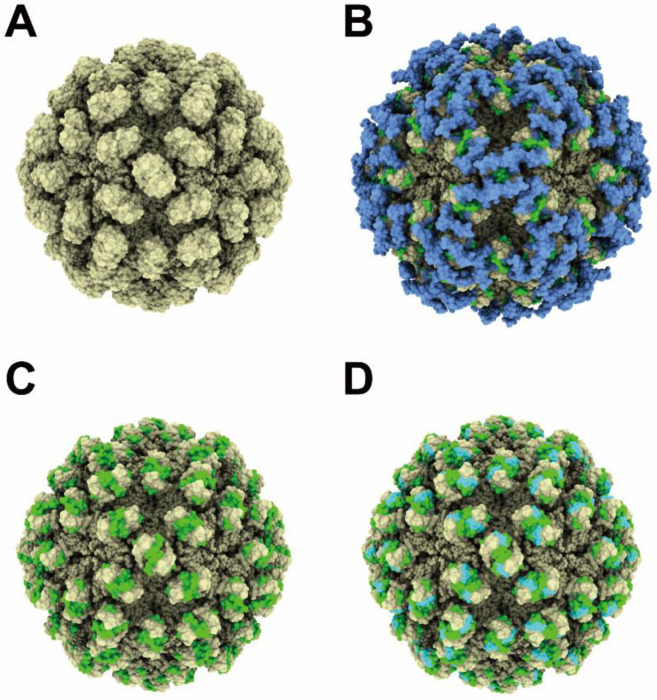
Protease-digested HEV virion structure. A predicted nonenveloped and nondigested HEV and nonenveloped and trypsin-digested virion structure. (A) Model-fitting image of the previously reported X-ray model of *T* = 3 VLP (PDB ID: 3IYO). The V470-A606 is replaced by the V470-A606 of the AlphaFold2-predicted ORF2 structure. (B) Superimposed image of 3IYO and the AlphaFold2-predicted ORF2 structure. The V470-A606 is replaced by the V470-S660 of the AlphaFold2-predicted ORF2 structure. The H6225-antibody-recognizing region, G551-R578, and the C-terminal region cleaved by trypsin V579-S660 are highlighted in green and blue, respectively. (C) The H6225-antibody-recognizing region, G551-R578, is highlighted in green. (D) The G551-R578 region and the V579-L601 region, which may be retained on the surface of fecal HEV or HEVcc, after treatment with detergent and trypsin, because of the interaction between the two β-strands (579–582; and 595–600) and other β-sheets (492–495 and 568–572; and 470–476 and 546–549, respectively), are highlighted in green and cyan, respectively.
